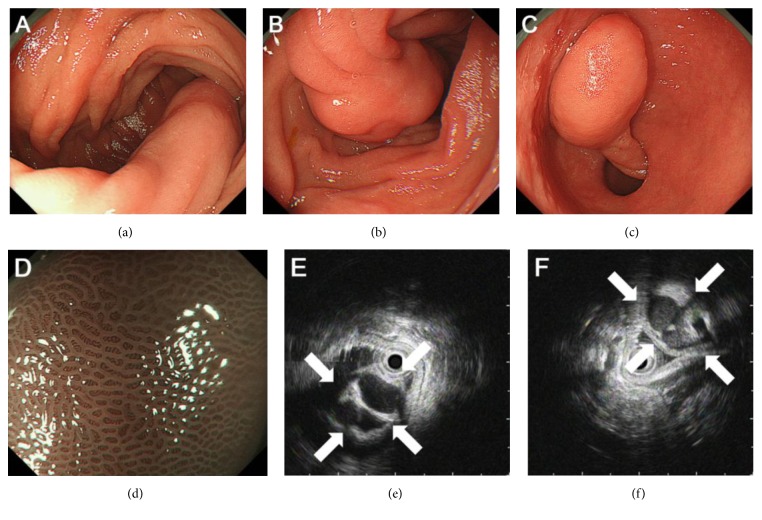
Endoscopy images. Esophagogastroduodenoscopy results showed a pedunculated polyp measuring approximately 20 mm ((a), (b)). The polyp was easily extended to the stomach by traction with biopsy forceps (c). Magnifying observation with narrowband imaging revealed that the surface of the polyp was entirely covered with intact duodenal mucosa (d). Endoscopic ultrasonography showed multiple cystic structures ((e), (f), arrows).
